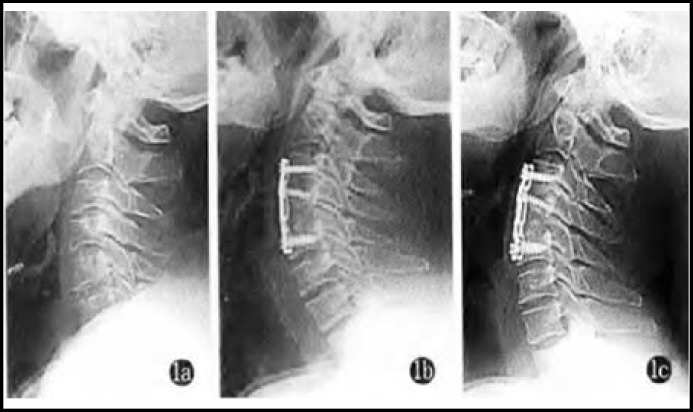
Mr. Wang, male, 62 years old, T_1_ segment. a: Preoperative X-ray film disclosed height of the injured vertebral body and Cobb angle; b: height of the injured vertebral body and Cobb angle immediately after central corpectomy; c: height of the injured vertebral body and Cobb angle three months after surgery (satisfactory maintenance and bone fusion).
